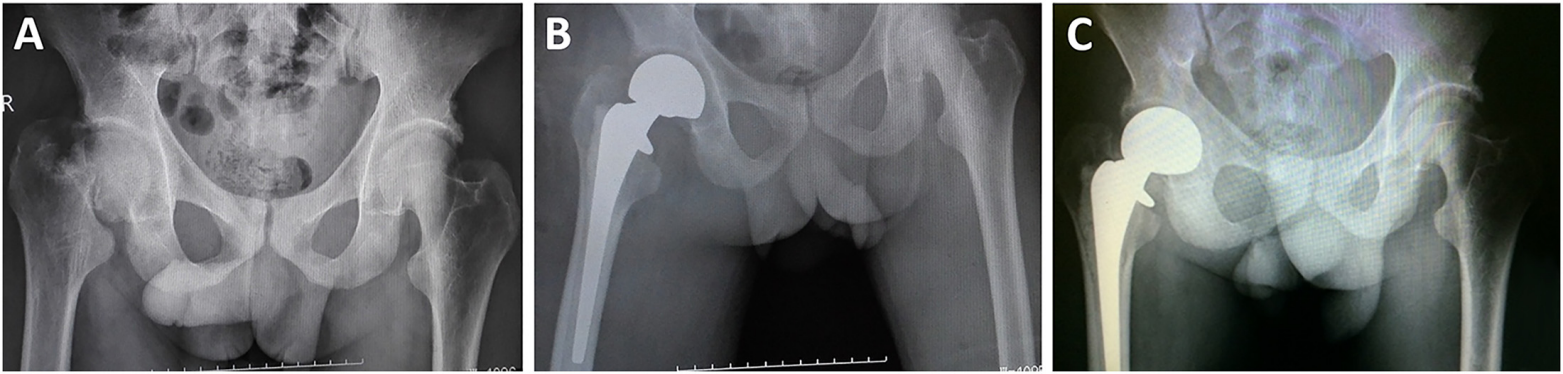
X-ray images of the right femoral neck fracture in a 72-year old male patient with chronic renal failure before and after hemiarthroplasty. (A) Preoperative imaging shows compression fractures on the femoral neck of the right hip and shortening deformity of the femoral neck. (B) Representative images taken 6 months after right femoral head arthroplasty. (C) At 24 months of follow-up, the prosthesis was in the correct position. On subsequent X-ray images, there were no further changes.
